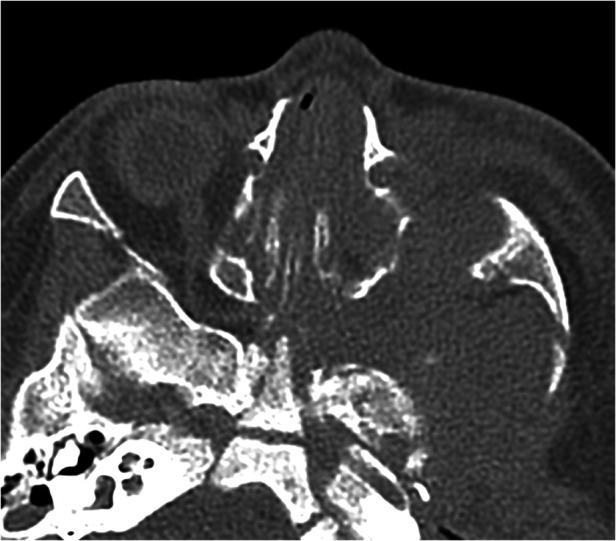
Axial CT scan of the paranasal sinuses shows a destructive inflammatory process of the left pterygopalatine fossa and widening of the foramen.
